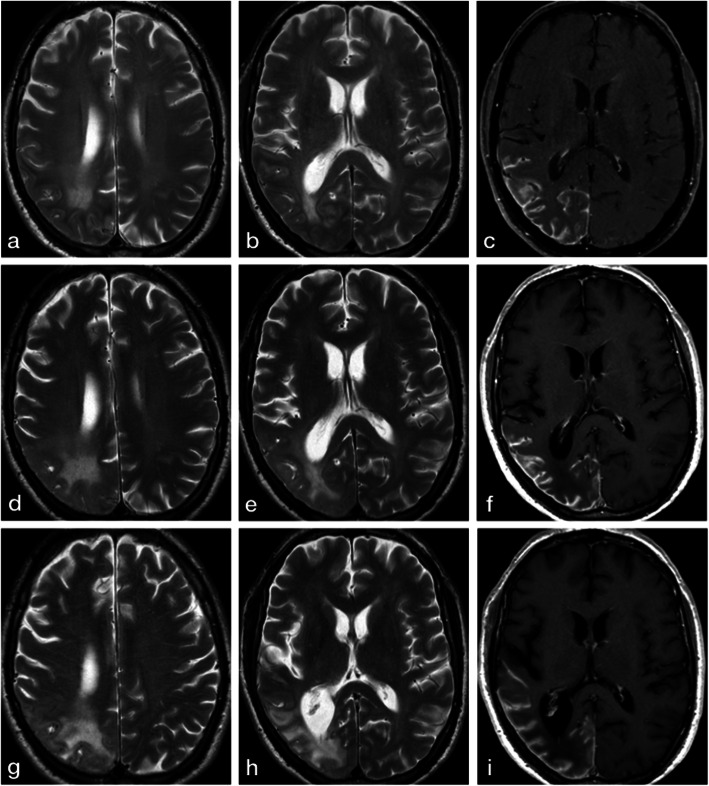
MRI brain demonstrating evolution of neuroradiological findings in the right temporo-parieto-occipital region at six months (a-c), one-year (d-f) and two years (g-i) follow-up. Notice the temporal progression of hyperintense signal changes in the subcortical white matter, subsequent cortical atrophy and ex-vacuo dilation of lateral ventricle in axial T2-weighted Turbo Spin Echo (TSE) images (a-b, d-e, g-h) and stable pial enhancement in axial T1-weighted Spin Echo (SE) images after gadolinium injection (c, f, i)
